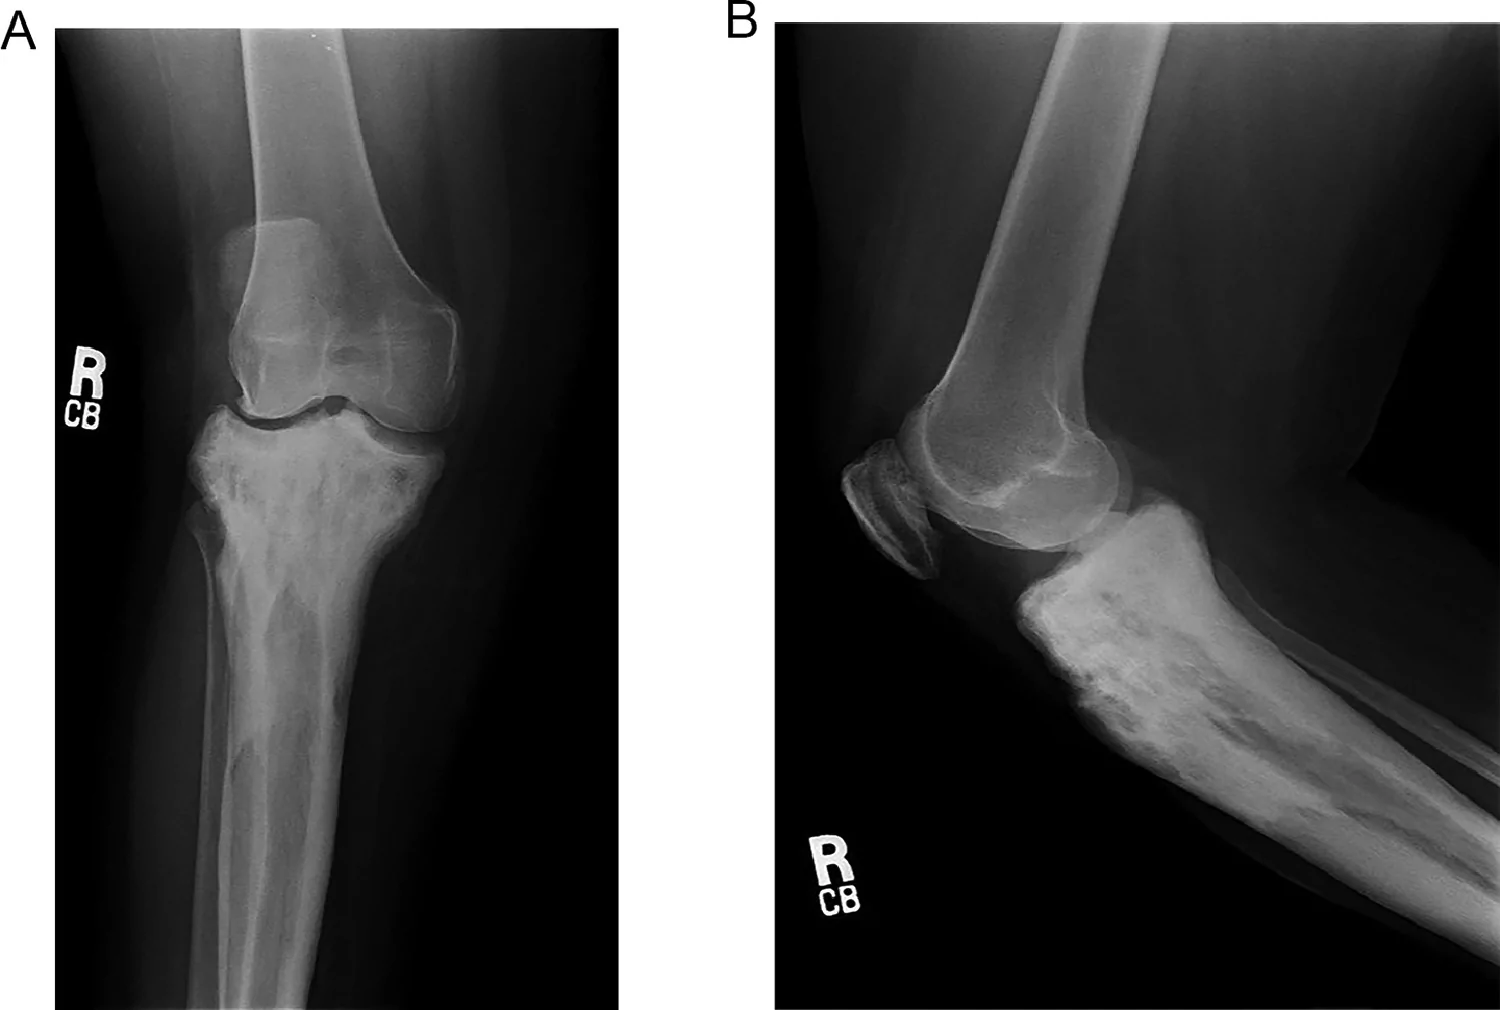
AP (A) and lateral (B) radiographs of the right knee demonstrate diffuse cortical thickening of the tibia as well as trabecular thickening/sclerosis of the proximal tibia including the tibial plateau, consistent with Paget’s disease of bone.
